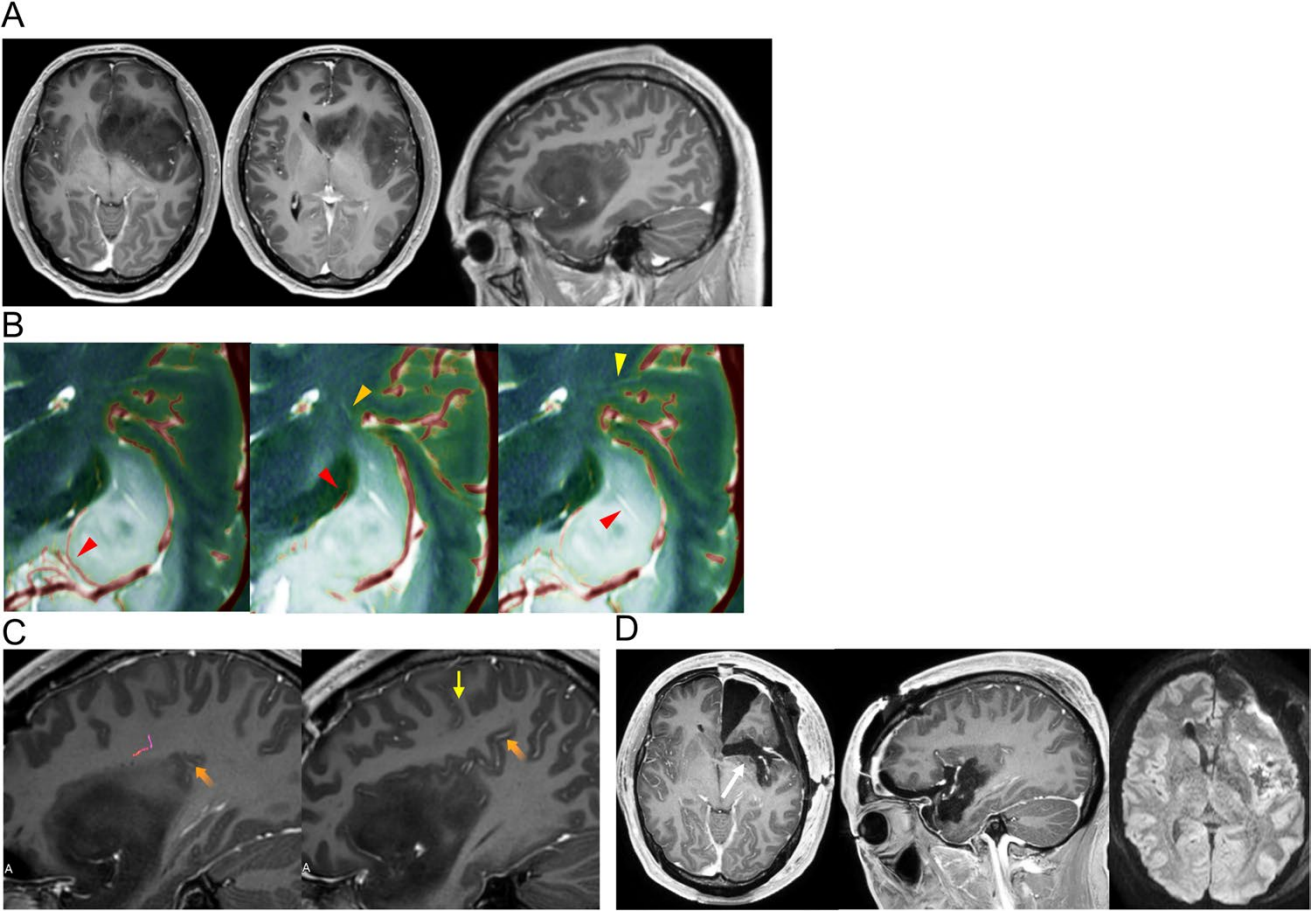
Preoperative assessment of the anatomical association between the long insular arteries (LIAs), long medullary arteries (LMAs), and lenticulostriate arteries (LSAs) and the tumor and postoperative magnetic resonance (MR) imaging in Case 2. A Preoperative axial (left and middle panel) and sagittal (right panel) T1-weighted MR imaging after the administration of gadolinium (Gd-T1WI) showing the lesion at the left subcallosal area and insula with the small enhanced area. B The coronal slice with LSAs (all panels), LIAs (middle panel), and LMAs (right panel) showing that the distal LSA (red arrows in the middle and right panel), LIA (orange arrowhead), and LMA (yellow arrow) were located outside from the hyperintense lesion. However, proximal LSA (red arrowhead in the left panel) was encased in the lesion. C Sagittal Gd-T1WI showing the origin of the LIA (orange arrow in the left panel) and LMA (orange arrow in the right panel), as shown in Fig. 2C. The yellow arrow indicates the central sulcus. The LIA and LMA originated from the posterior region of the insula and the sulcus within the supramarginal sulcus, respectively. D Postoperative axial (left panel) and sagittal (middle panel) Gd-T1WI and diffusion-weighted MR imaging (right panel) showing the subtotal resection of the lesion without infarction due to perforating injury. The arrow indicates the residual region at the anterior perforating substance where the LSA was encased by the tumor
